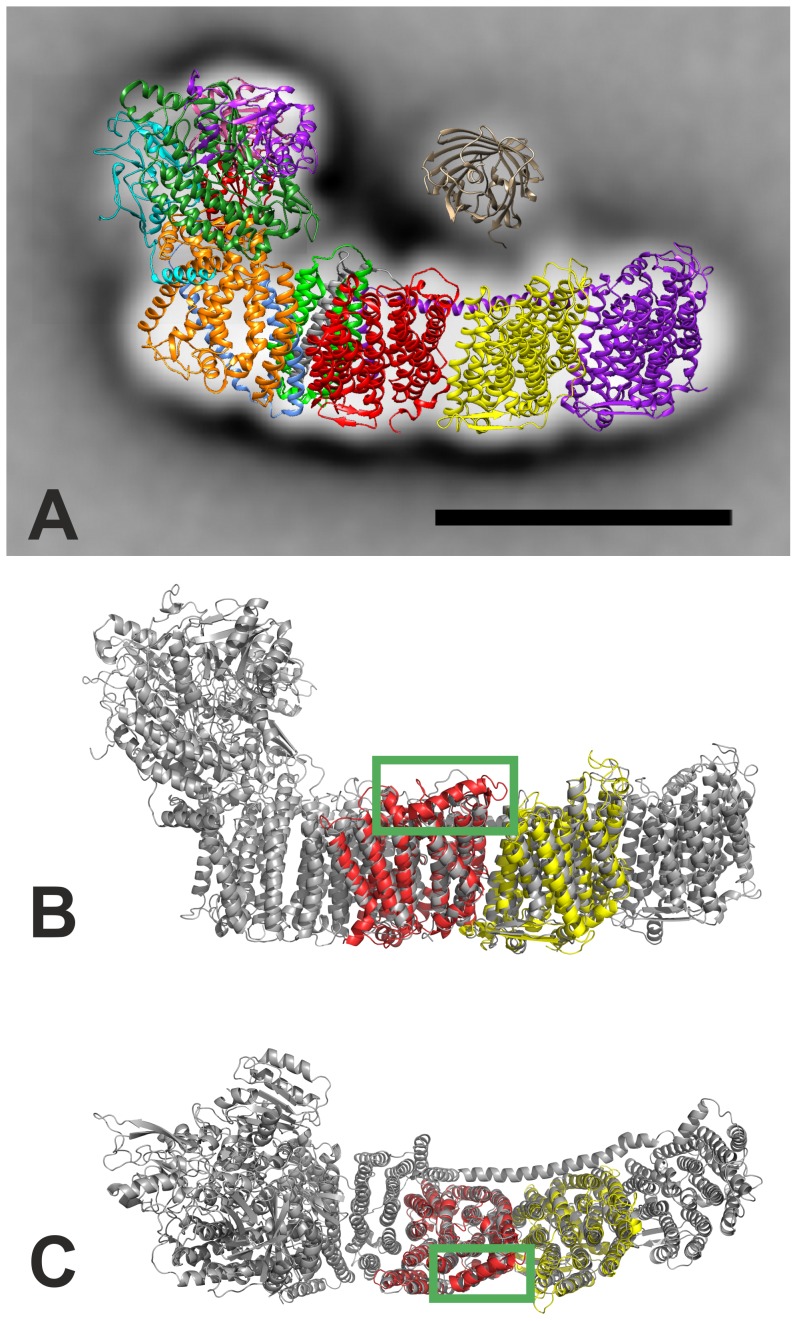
Localization of the NdhP subunit. A: Fitting of the NDH-1L projection map with sfGFP [20] (PDB code: 2B3P) to the X-ray structure of NDH-1 from *T. thermophilus*
